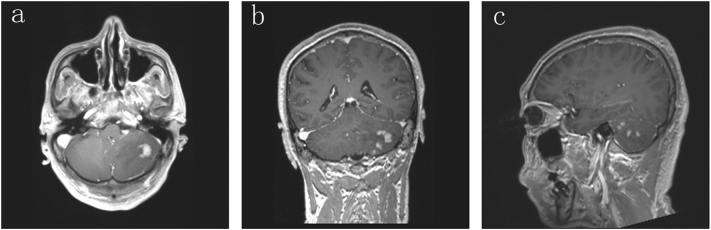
Magnetic resonance imaging enhancement is very evident in the cerebellum (bilateral), with multiple masses observed, and the largest mass is localized in the left cerebellum.
